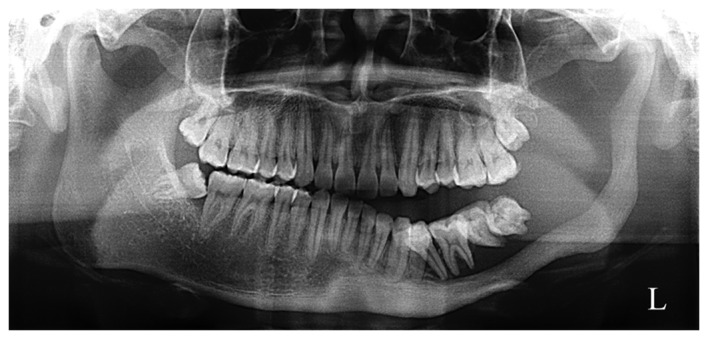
Panoramic radiograph revealing severe bone resorption of the left mandibular body and ramus.
